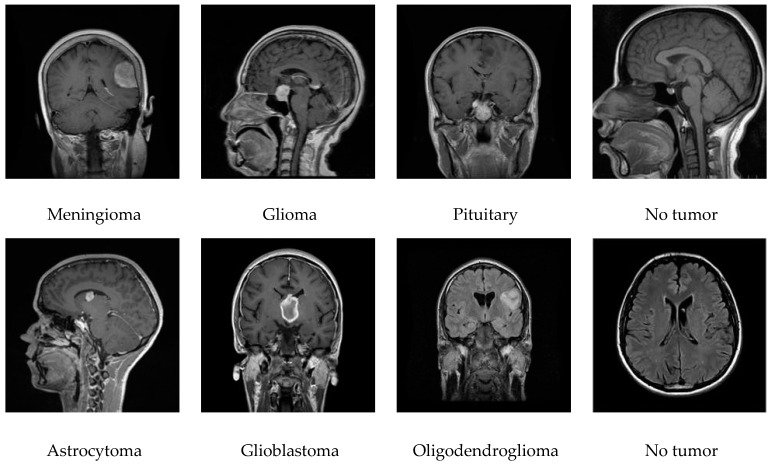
Sample MRI images in the dataset.
